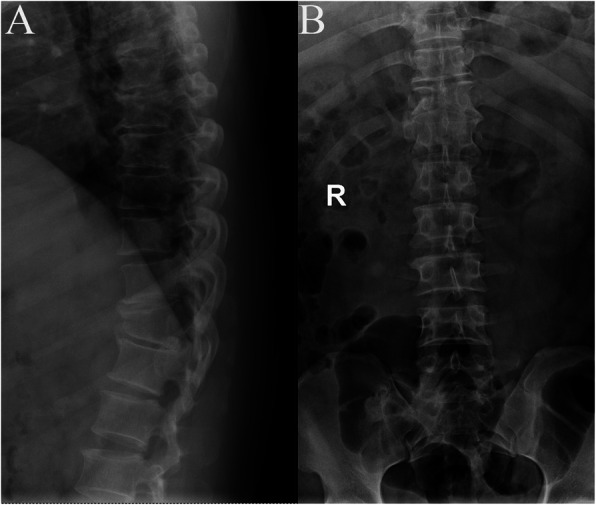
Plain radiograph of the thoracic vertebrae (a, lateral view) shows anterior wedging of T11, which could be easily confused for a compression fracture. An anteroposterior view (b) shows a symmetrical defect with corticated margins in the T11 vertebral body
